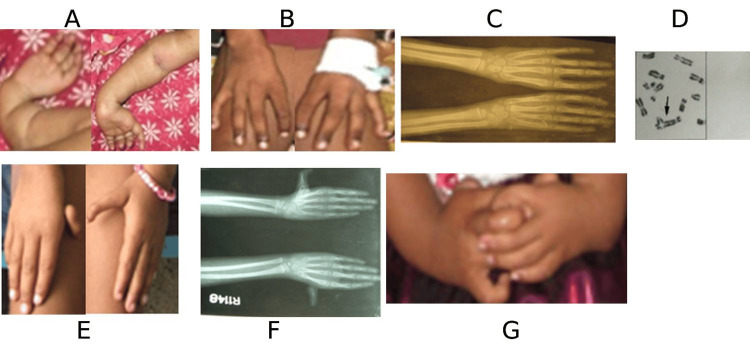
Physical abnormality and chromosomal aberration study A: cropped picture hands of patient P6 showing four digits with an absent thumb in both hands; B: cropped picture hands of patient P7 showing four digits with an absent thumb in both hands; C: an X-ray image of both hands of patient P7 showing absence of thumb in both hands; D: chromosomal aberration of patient P8; E: cropped picture hands of patient P10 showing anomaly in both thumbs; F: an X-ray image of both hands of patient P10 showing triphalangeal thumb in the left and absent first metacarpal in the right hand; G: cropped picture hands of patient P11 showing bilateral radial ray defect and absent radius on the left side
